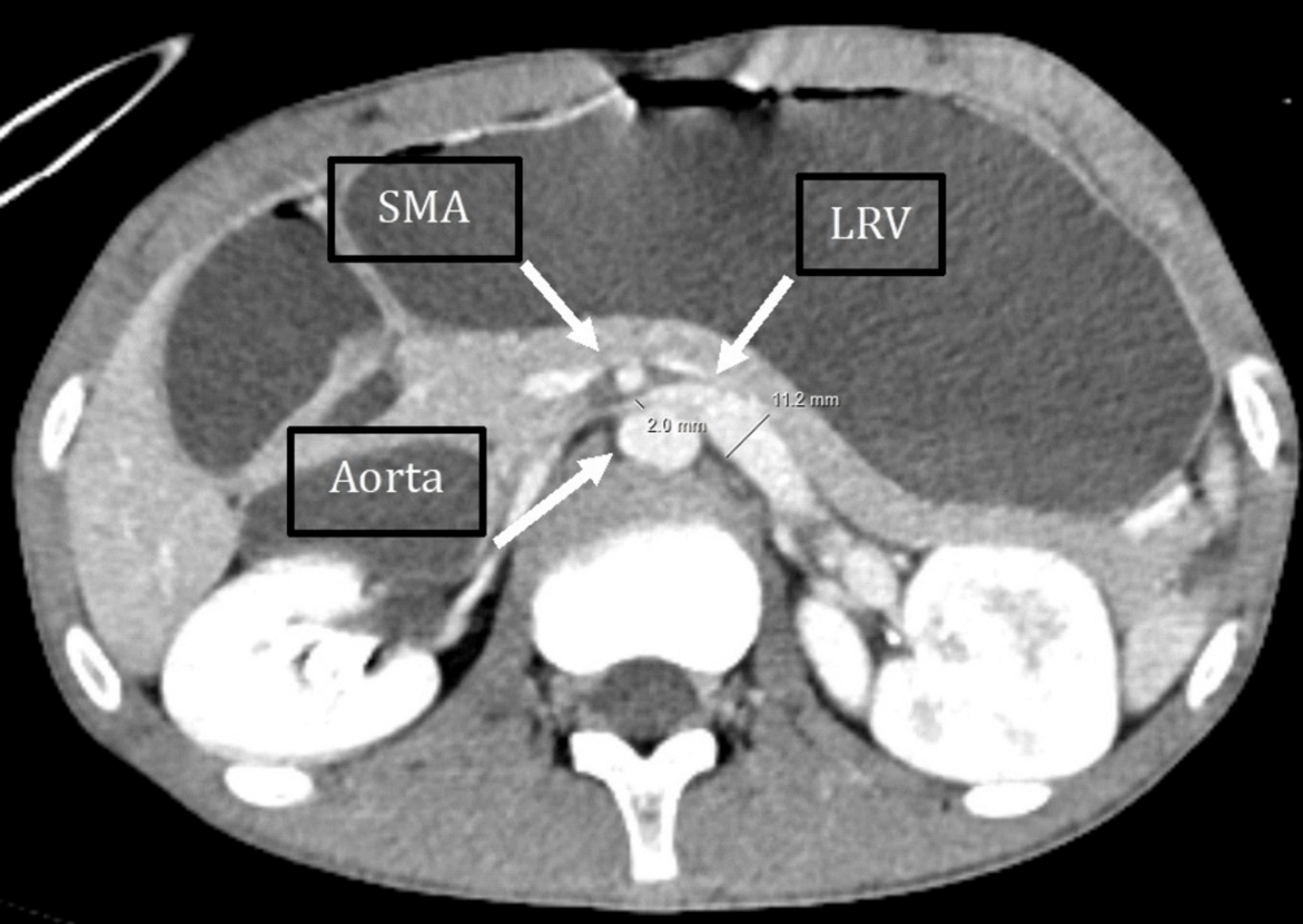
Contrast-enhanced computed tomography (CT); axial reconstruction in the portal venous phase shows narrowing of the left renal vein (LRV) at the level of the aortomesenteric clamp, with proximal venous dilation. SMA: superior mesenteric artery
